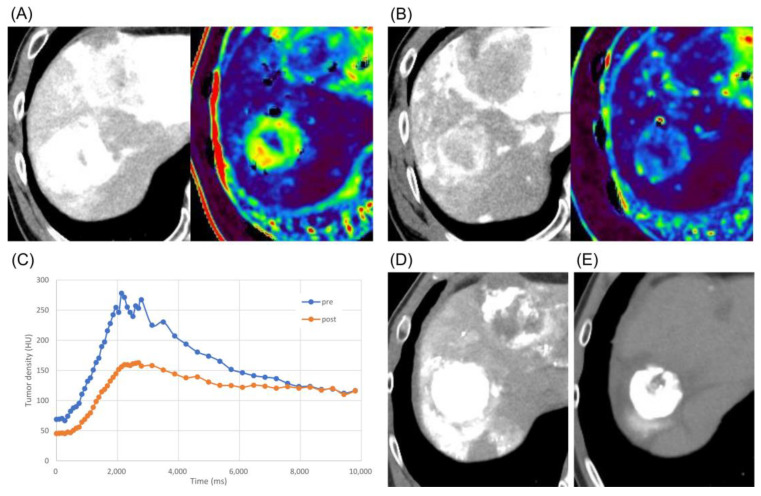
Case 2: quantification of tumor vascularity. (A) 4D-CTHA and perfusion map just before the initiation of lenvatinib. Arterial blood flow (ABF) to the tumor was 288.2 mL/min/100 mL. (B) 4D-CTHA and perfusion map after lenvatinib administration for 4 days. The ABF decreased to 122.6 mL/min/100 mL, which was 42.5% of the value before lenvatinib administration. (C) Time intensity curves pre- and post-treatment: a decrease in intratumoral density was observed post-treatment compared to pre-treatment. (D) Plain CT immediately after cTACE: lipiodol accumulated well in the tumor. (E) Plain CT after 5 months: the tumor had decreased in size with persistent complete lipiodol retention in the lesion.
